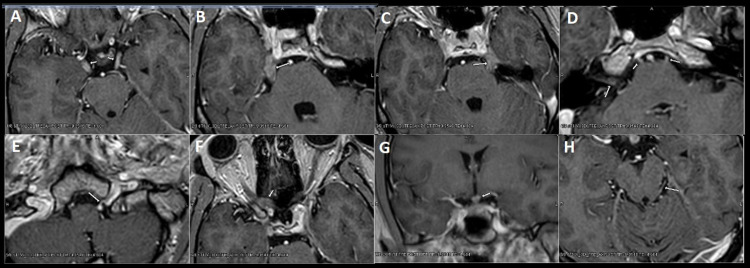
MRI brain images demonstrating multiple cranial nerve thickening and enhancement Axial post-contrast MRI sequences depicting thickening and enhancement of the (A) bilateral optic tracts, (B) right trigeminal nerve, (C) left trigeminal nerve, (D) bilateral abducens nerves and right facial nerve, (E) left hypoglossal nerve, (F) right optic nerve, (G) infundibulum, and (H) left trochlear nerve.
